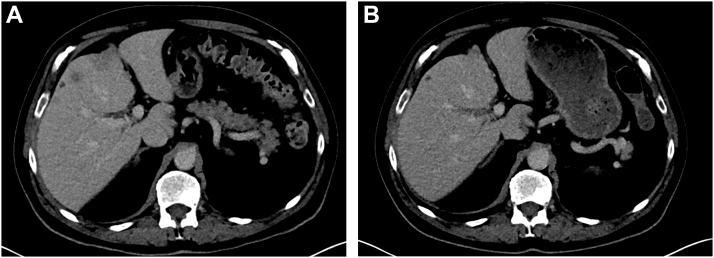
CT axial sections demonstrating response to therapy in liver and pleural disease between (*A*) cycle 1 day 1 T-DXd and (*B*) after 4 cycles. CT, computed tomography; T-DXd, trastuzumab-deruxtecan.
